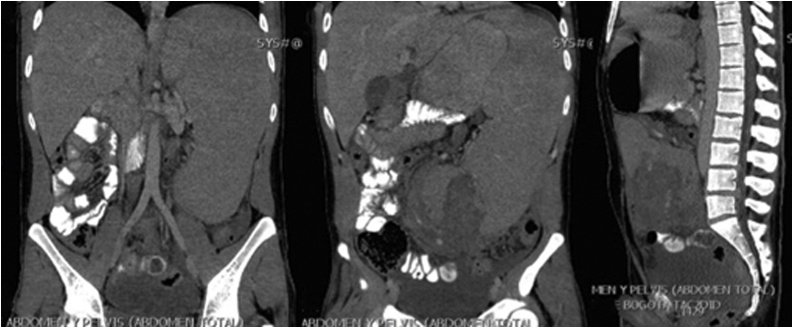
Abdominal CT scan coronal slices at abdominal aorta level (Left) Cecum (Middle) and midline sagittal slice (Right). Patient data was deleted.
